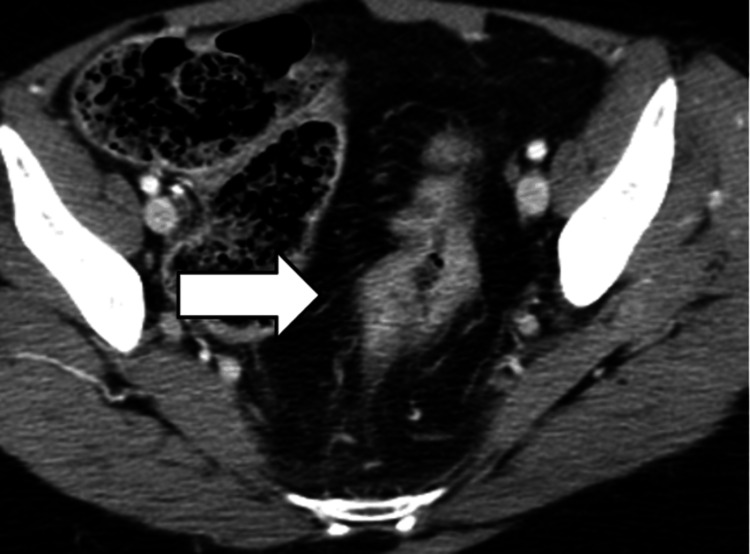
Mesenteric fibro-fatty proliferation. CT image shows mesenteric fibro-fatty proliferation (creeping fat) of the sigmoid mesentery in a patient with Crohn's disease (white arrow).
